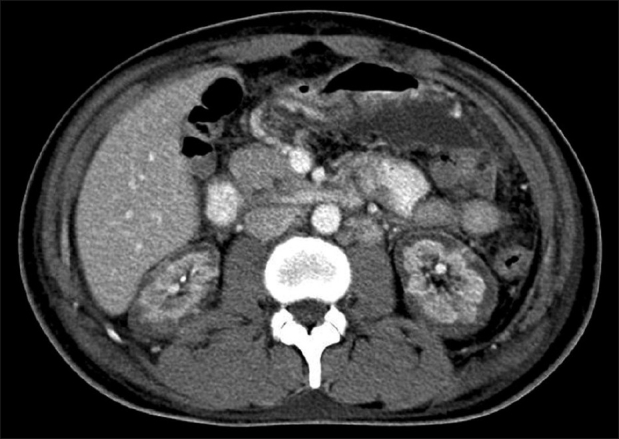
Contrast enhanced CT of the abdomen showing diffuse hypodense areas in the cortex surrounded by capsular enhancement in both the kidneys
